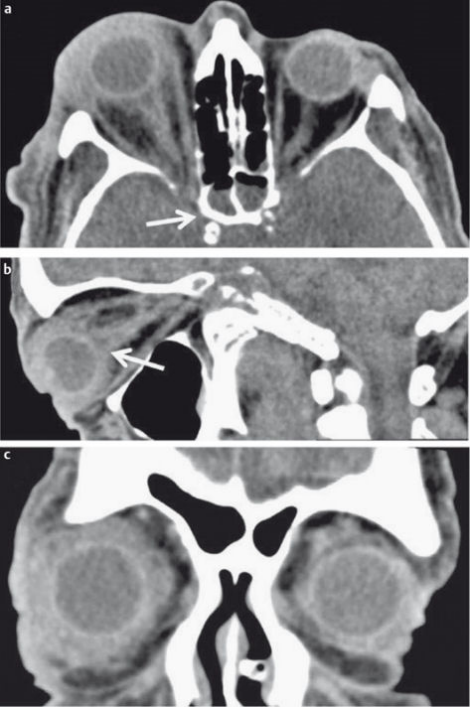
74-year-old diabetic patient with acute onset of nerve palsy of the 3^rd^, 4^th^ and 6^th^ cranial nerves on the right side and progressive “reddening” of the right eye and painful eye movement. a) Axial CT demonstrates inflammatory changes of the right sphenoid sinus (arrow). b) Sagittal reconstructed CT images demonstrate diffuse inflammatory changes of the globe and the optic nerve (arrow). c) Coronal reconstructed CT images demonstrate subtle inflammatory changes also on the left side. MRI (not shown) demonstrated thrombosis of the cavernous sinus on both sides, which was not detectable on CT. Surgery confirmed Candida infection.
